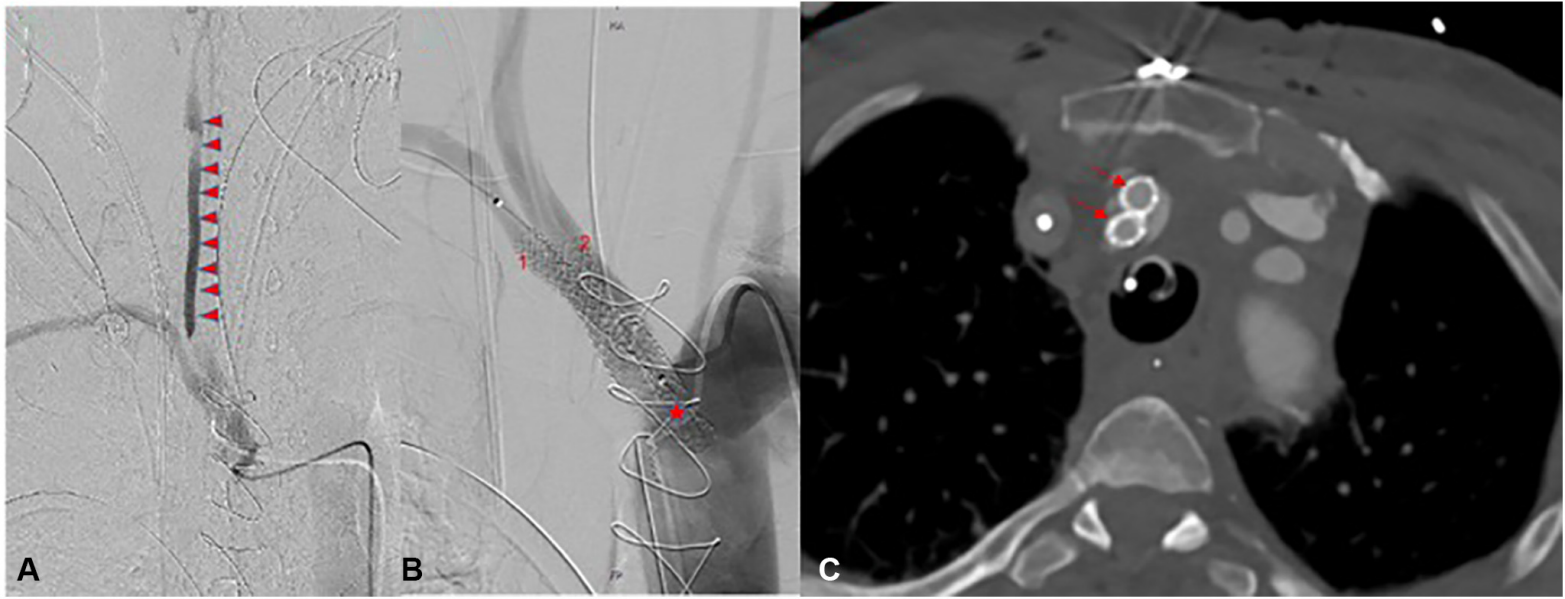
A, Angiographic imaging of the innominate artery shows retention of the contrast agent in the false lumen of the dissected common carotid artery (*arrowheads*). B, The dissected innominate artery and the right common carotid artery were treated with 2 kissing stents, which reached out into the aortic arch (*star*). The technique of the kissing stents ensures optimal distal perfusion of both the right subclavian and right carotid arteries and precludes compression or occlusion of one vessel through the other one. In addition, kissing stents allowed a safe closure of the supposed entry in the trunk, whereas vascular access in the true lumen was secured using ultrasound-guided puncture of the right carotid artery. C, Postinterventional computed angiography showing the 2 parallel stents in the innominate artery (*arrows*).
